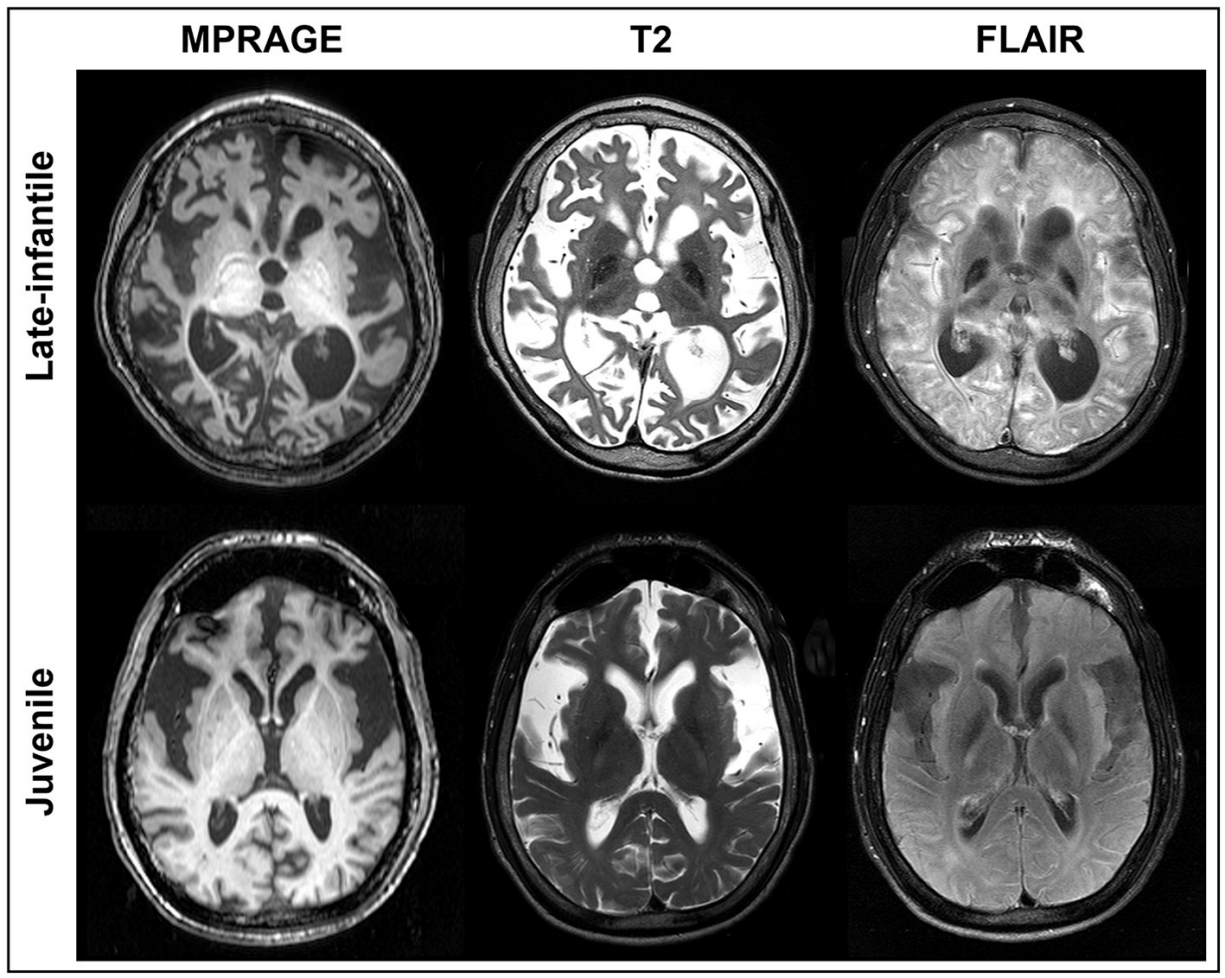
MRI of GM1 patients. Axial MPRAGE, T2, and FLAIR images are shown at the same level for a representative late-infantile (top) and juvenile (bottom) patient. While both cases demonstrate marked degeneration, the late-infantile patients were more severe, adding difficulty when generating regions of interest (ROIs). ROIs for each brain structure were determined based on the MPRAGE or 3D FFE images given the overall clarity of the structures.
